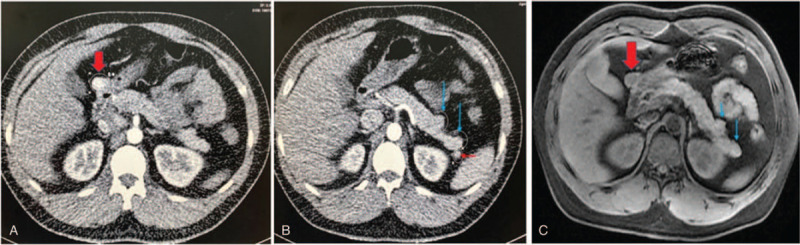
Pancreatic VPCT (A, B) and MRI (C) of the patient. (A)The thick arrow indicated the nodule on the right side of the gastric antrum (maximum cross-section of approximately 21 mm × 16 mm). (B)The thin arrows showed 2 small nodules in the tail of the pancreas (13 mm and 7 mm in diameter, respectively), and the short arrow showed a nodule in the hilum of the spleen. (C) The thick arrow indicated the nodule on the right side of the gastric antrum, and the thin arrows showed 2 small nodules in the tail of the pancreas.
